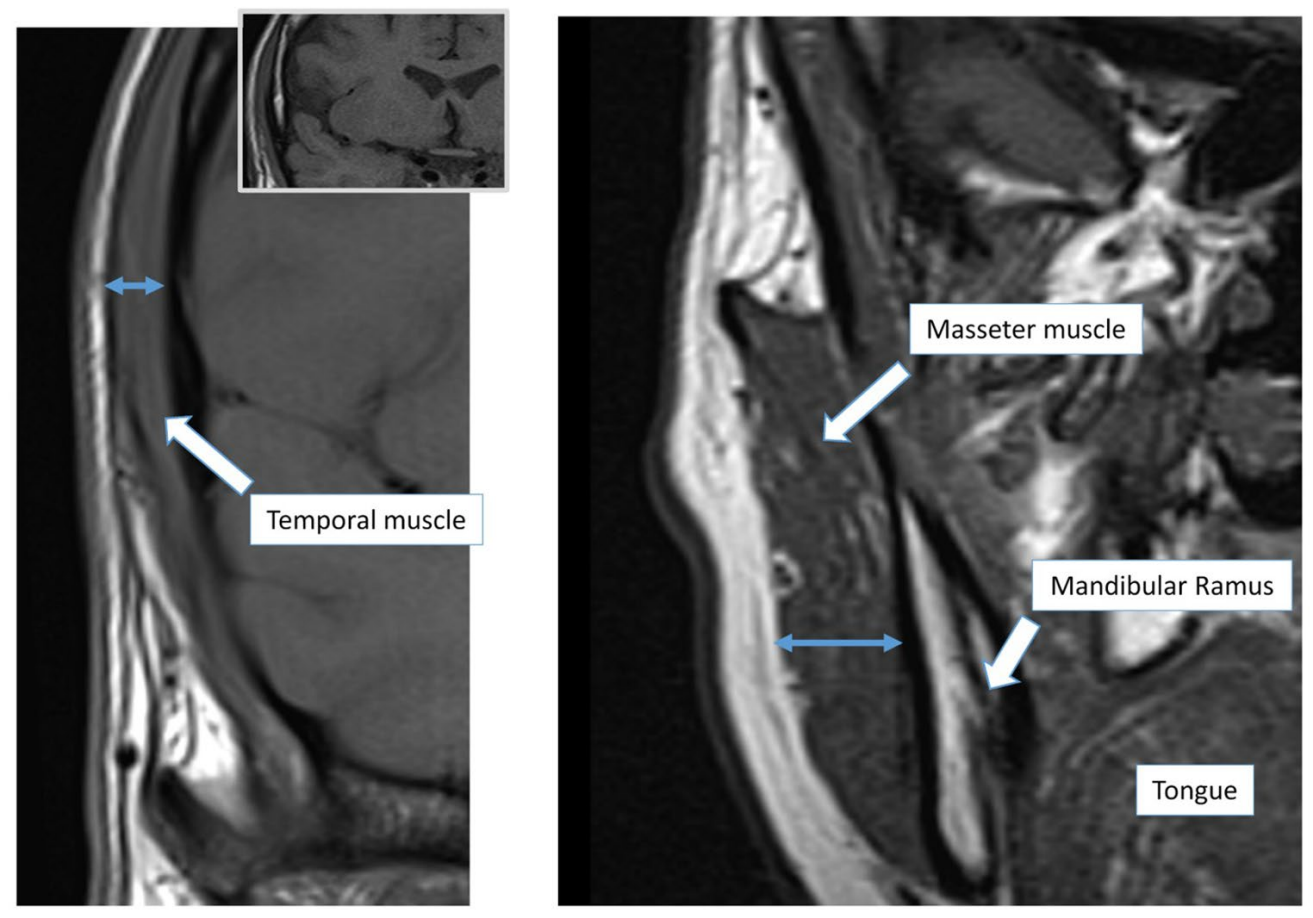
Examples of temporal (panel A) and masseter (panel B) muscle thickness measurements in two different patients on coronal T1-weighted MRI sequences (double headed arrow). *Abbreviations.* MRI, magnetic resonance imaging
